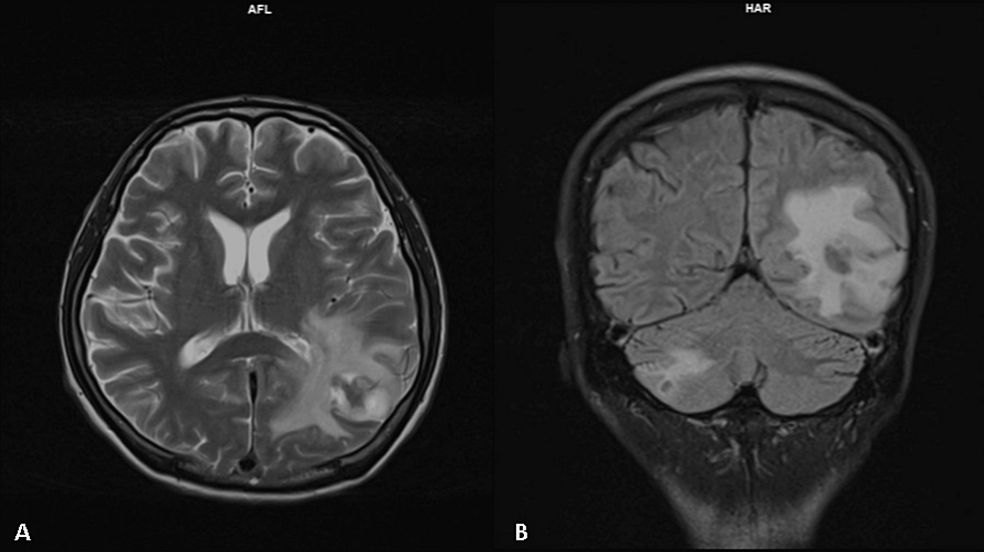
Magnetic resonance imaging of the brain suggestive of tuberculomas. Axial view (A) showing a well-defined nodular rim-enhancing white matter lesion with central non-enhancing hypointensity at the left parieto-occipital lobe measuring approximately 2.2 x 2.2 x 1.7 cm. There is marked perilesional white matter edema extending to the left temporal lobe. Minimal leptomeningeal enhancement is seen in the adjacent left cerebral hemisphere. The coronal view (B) shows another smaller lesion with similar nodular rim enhancement seen at the right cerebellar hemisphere measuring approximately 1.5 x 1.3 x 1.1 cm associated with minimal surrounding perilesional edema and adjacent leptomeningeal enhancement.
